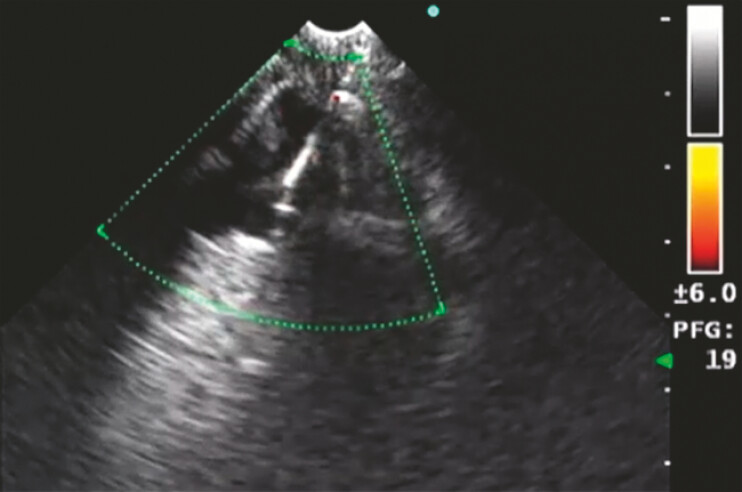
Ultrasound image showing common hepatic duct punctured by a 19G fine-needle aspiration needle.
